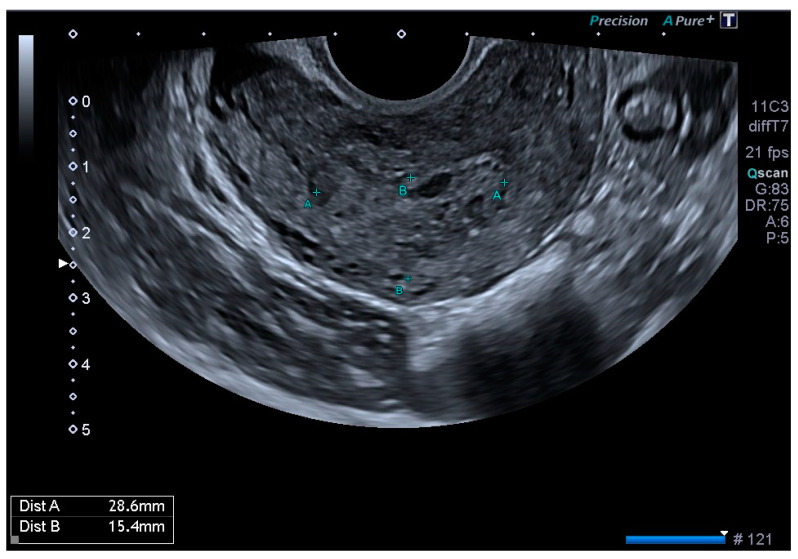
Transvaginal ultrasound with suspected uterine arteriovenous malformation.
